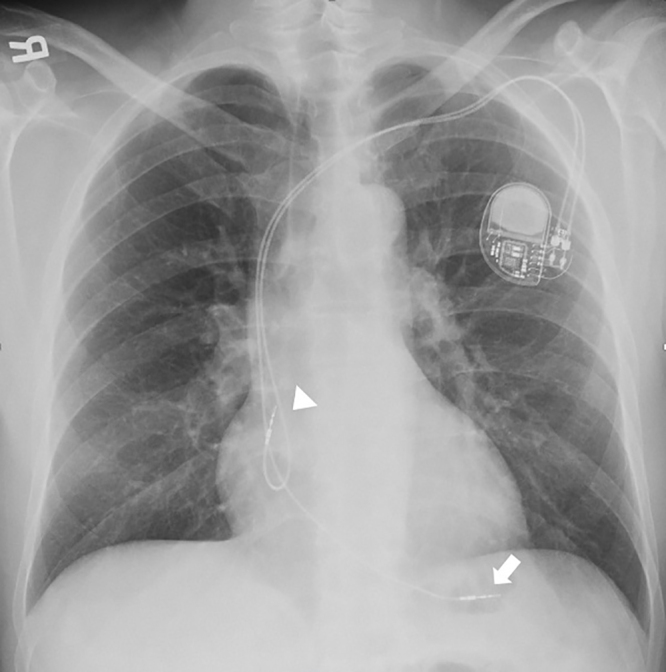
Chest radiograph revealing ventricular pacer lead located distally overlying the right ventricle apical area (arrow) and atrial pacer lead within right atrium area (arrowhead).
